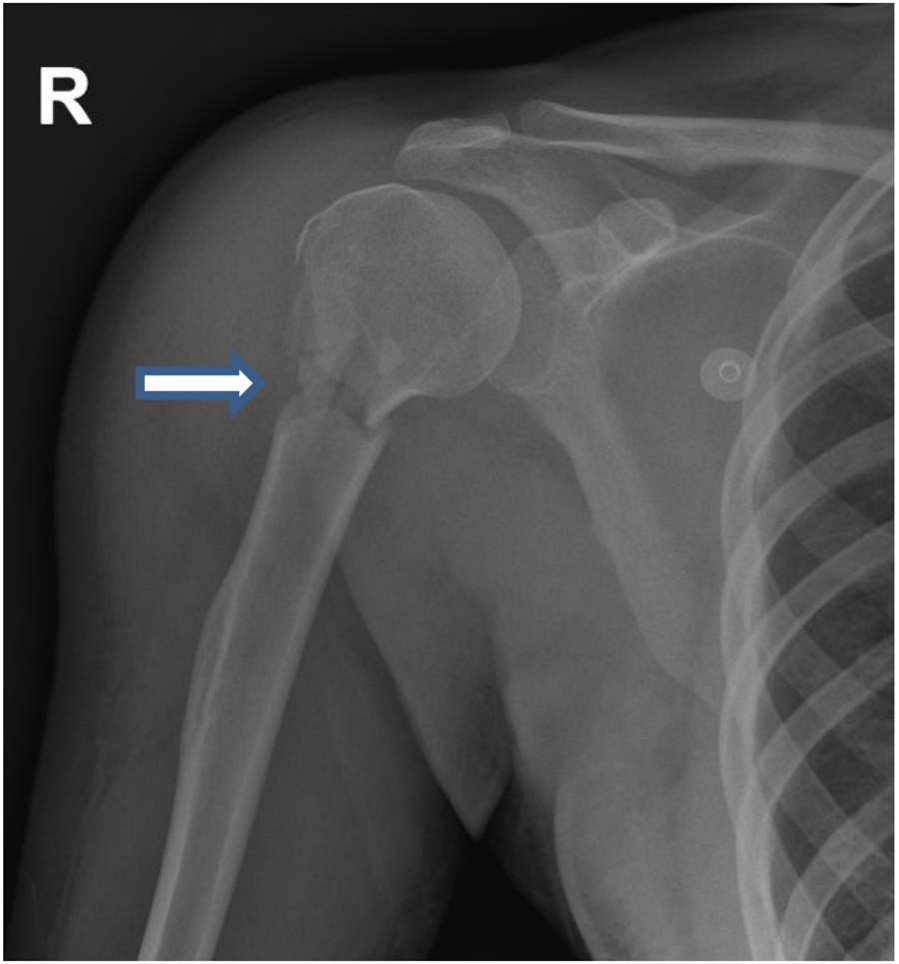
Upper limb X-ray revealed fracture of right Humerus.
